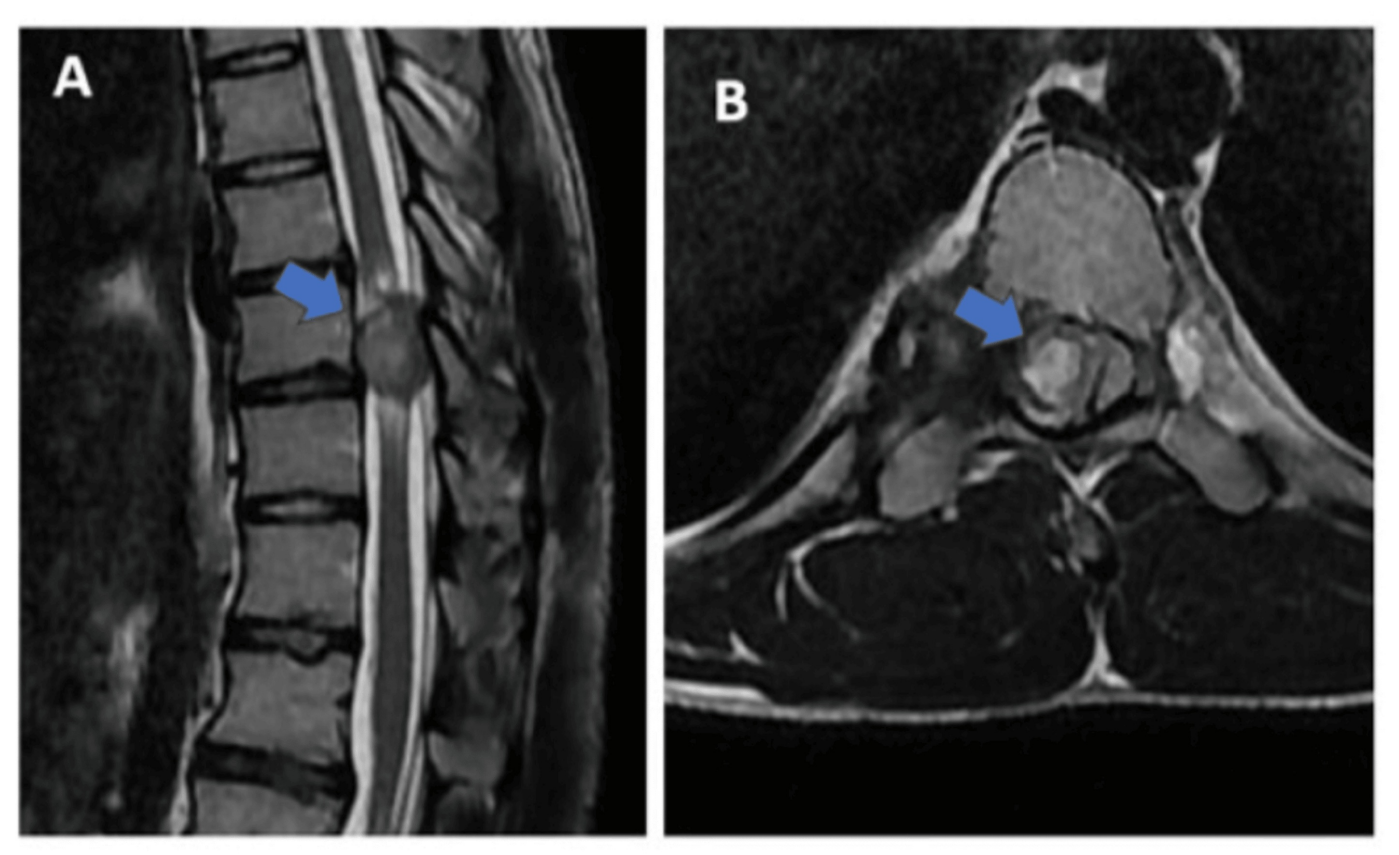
MRI spine showing hypointense lesion in the spinal canal. (A) Blue arrow showing the lesion in the spinal canal with cord edema. (B) Blue arrow showing the lesion arising from the posterior aspect of the ninth rib extending into the D9-D10 neural foramen, compressing the exiting nerve root and displacing the spinal cord towards the left side, causing compression. MRI: magnetic resonance imaging.
